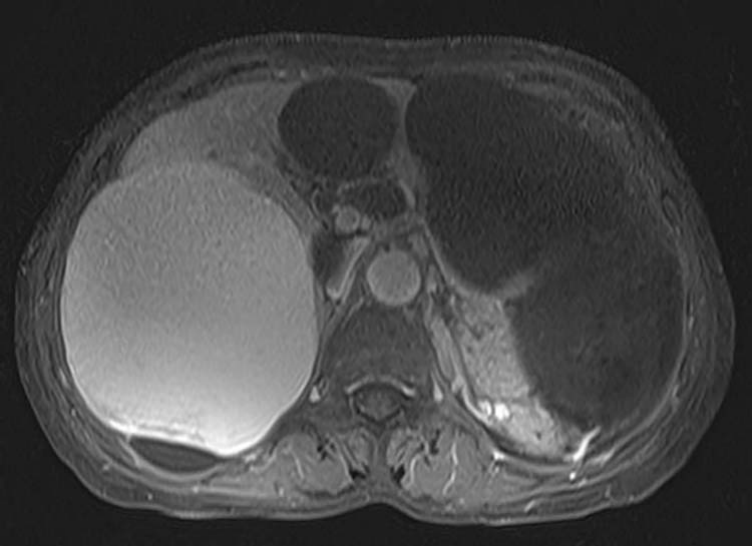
Preoperative T1-weighted magnetic resonance imaging showing different findings in the right and left lobe cysts.
